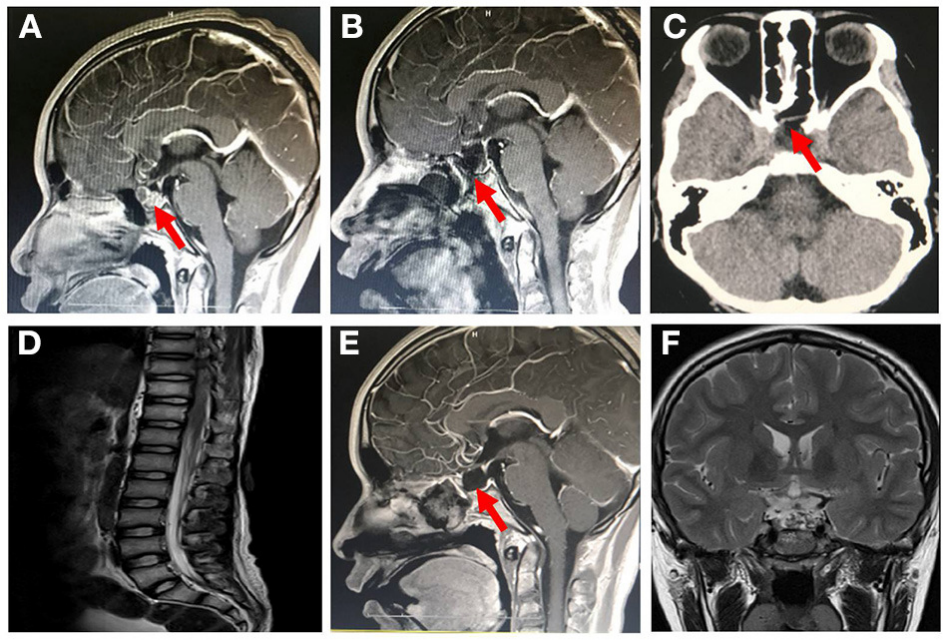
Brain magnetic resonance imaging (MRI) and computed tomography (CT) image after patient admission. (A) Sagittal enhanced MRI of pituitary showed enlarged sella turcica with a mass of about 1.2 cm × 1.6 cm × 1.4 cm in it, pituitary stalk extension to the right side and optic chiasm compression pituitary (January 25, 2019). (B) Sagittal enhanced MRI of pituitary showed that the craniopharyngioma was completely removed, and the skull base was incompletely covered by mucosal flap (January 29, 2019). (C) On day 47 of admission (March 12, 2019), nonenhanced skull CT scan revealed that the cerebrospinal fluid leakage was successfully repaired owing to the skull base reconstruction with mucosal flap. (D) Whole-spine MRI revealed no obvious adhesion in the spinal canal after 4 days of polymyxin B treatment (March 19, 2019). Follow-up pituitary MRI revealed (E) no recurrence of tumor, well healed mucosal flap in skull base, and (F) no obvious hydrocephalus (January 16, 2020).
